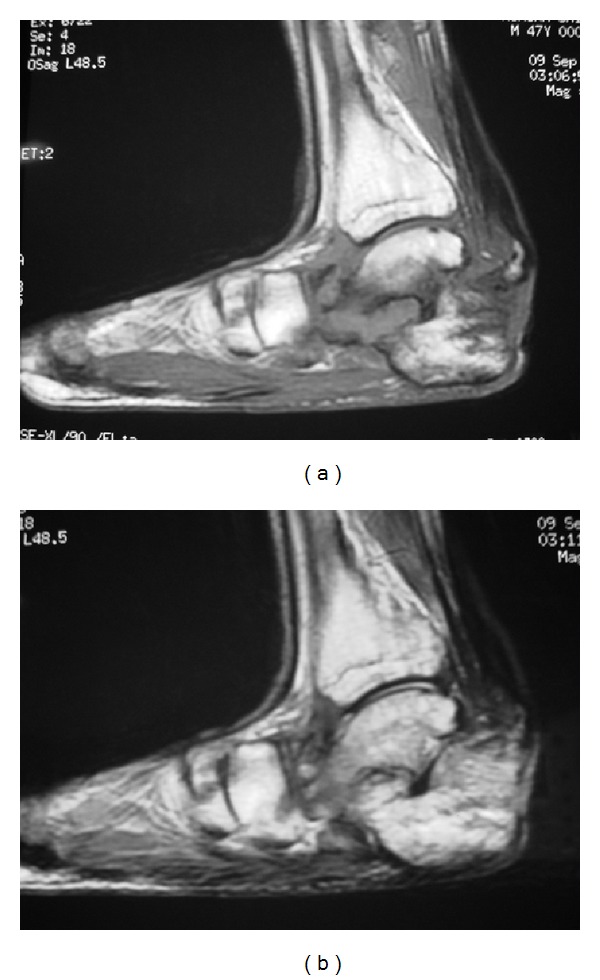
Magnetic resonance imaging of one year after operation. (a) T1-weighted sagittal imaging shows diffuse high signal intensity in posterior calcaneus. (b) T2-weighted sagittal imaging shows diffuse high signal intensity in the same region.
